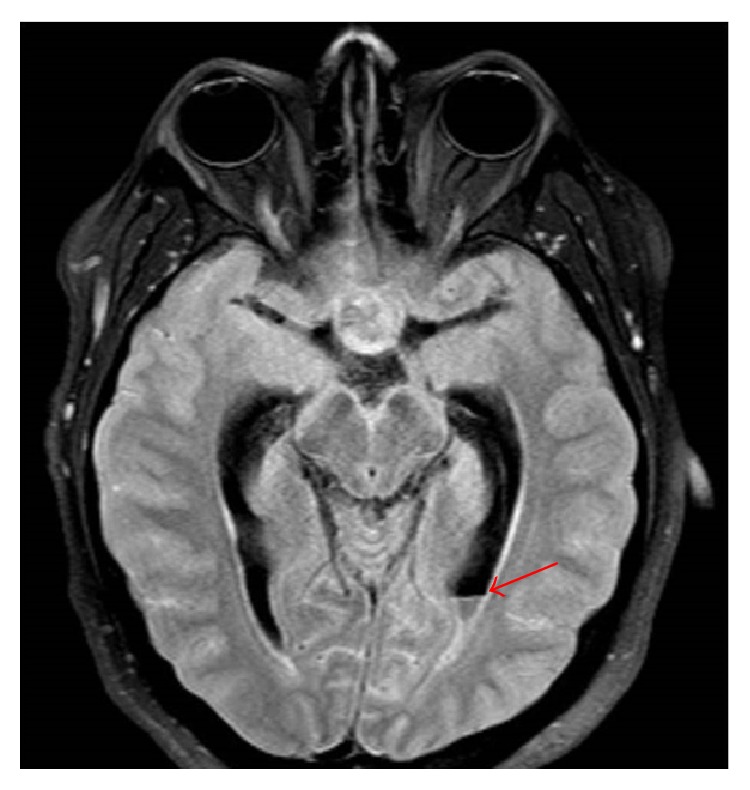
T1 AX FLAIR (brain MRI with and without contrast). Red arrow indicating debris layering in the left ventricle.
